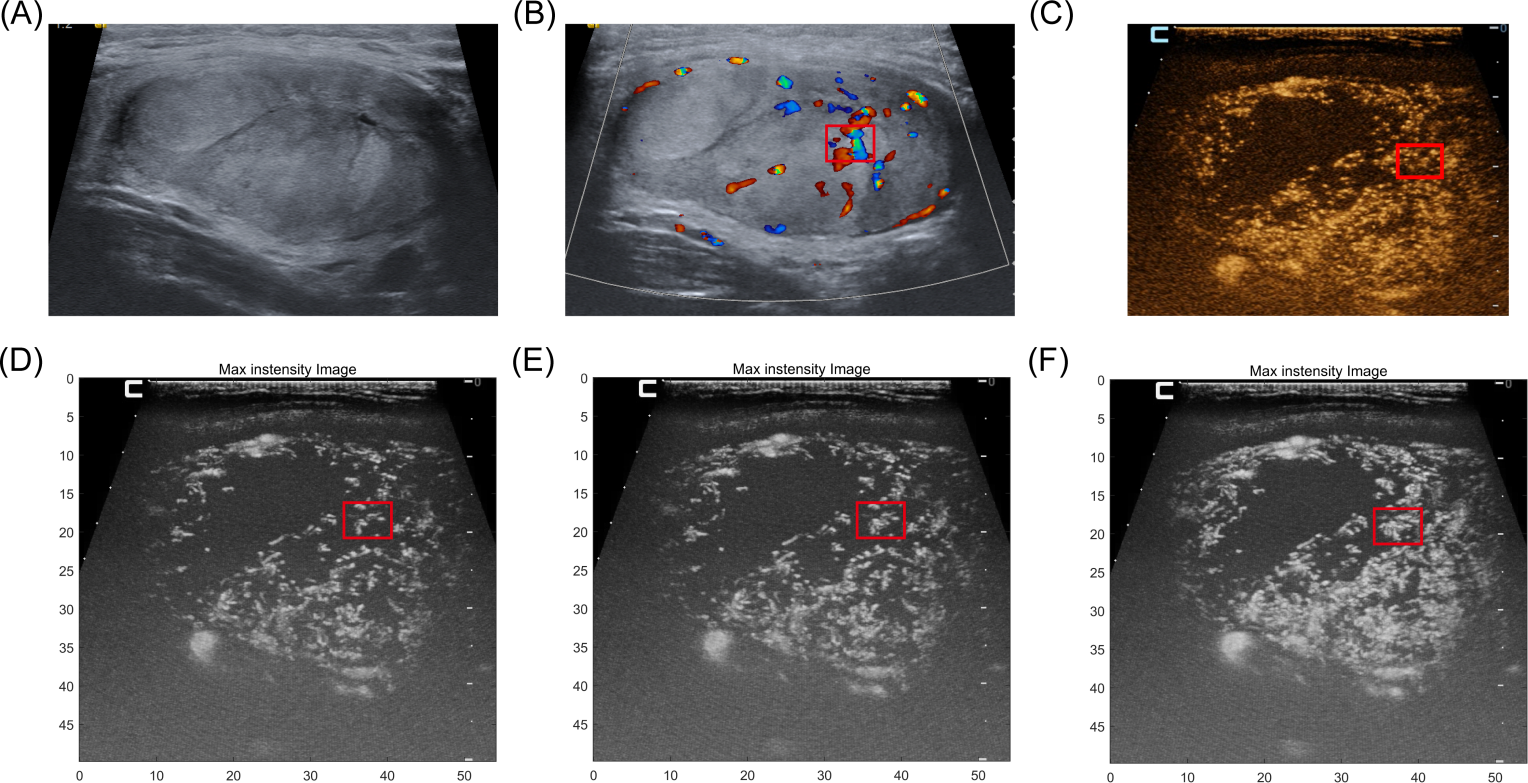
A 36-year-old man with FTC proven by pathology. (A) B-mode ultrasound of FTC. (B) color Doppler image of this case. only several thick blood vessels are displayed. (C) For the CEUS image, the microbubble just be record as a brief glimpse, the continuous vascular structure of the area is not displayed. (D–F) The microvascular shape with the typical firework-like feature, as shown in the red box but the information displayed can be continuously obtained by MIP sequences.
